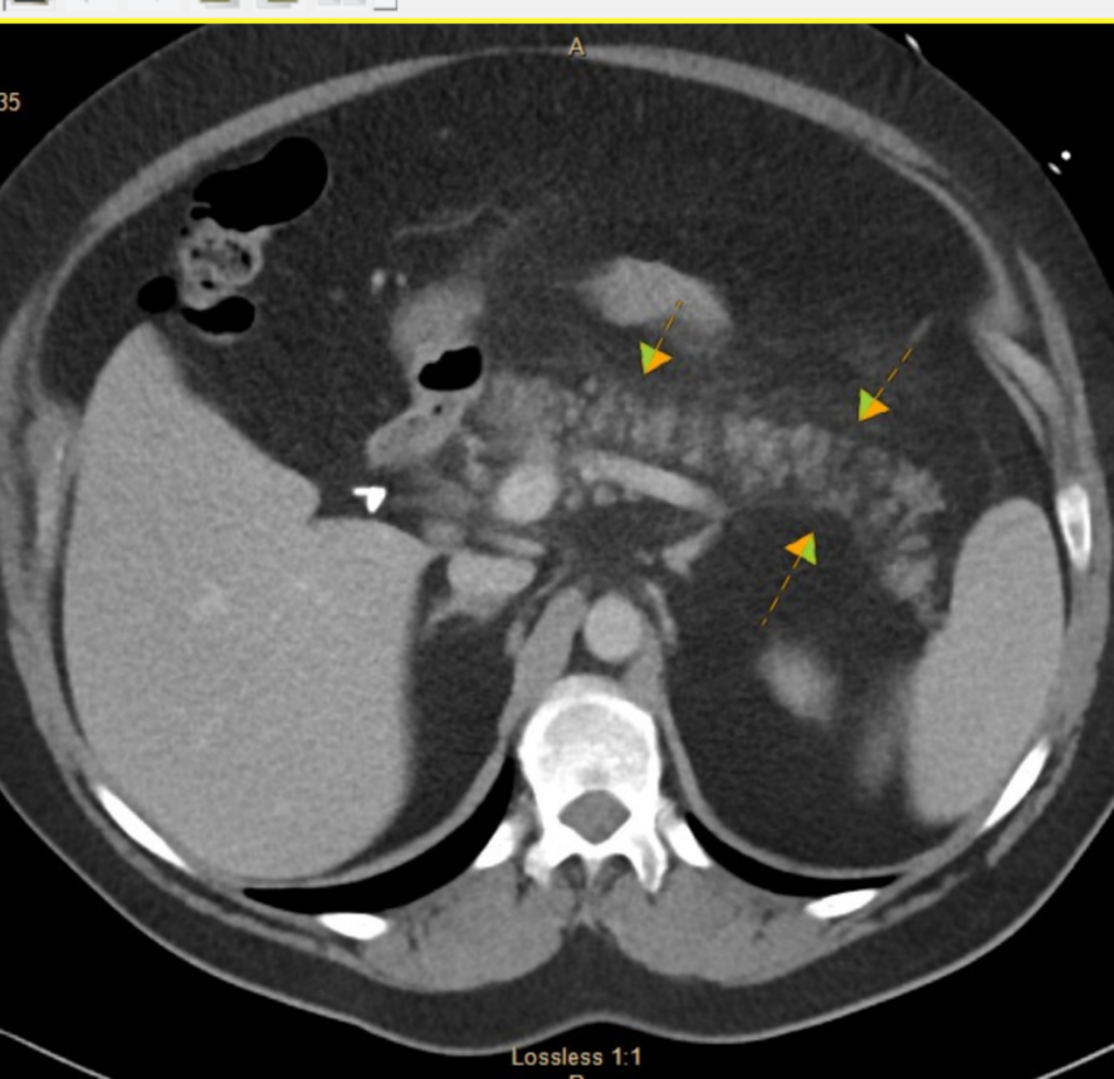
CT scan showing the pancreas appears diffusely edematous and there is some induration of the fat around the pancreas suggestive of mild pancreatitis.
